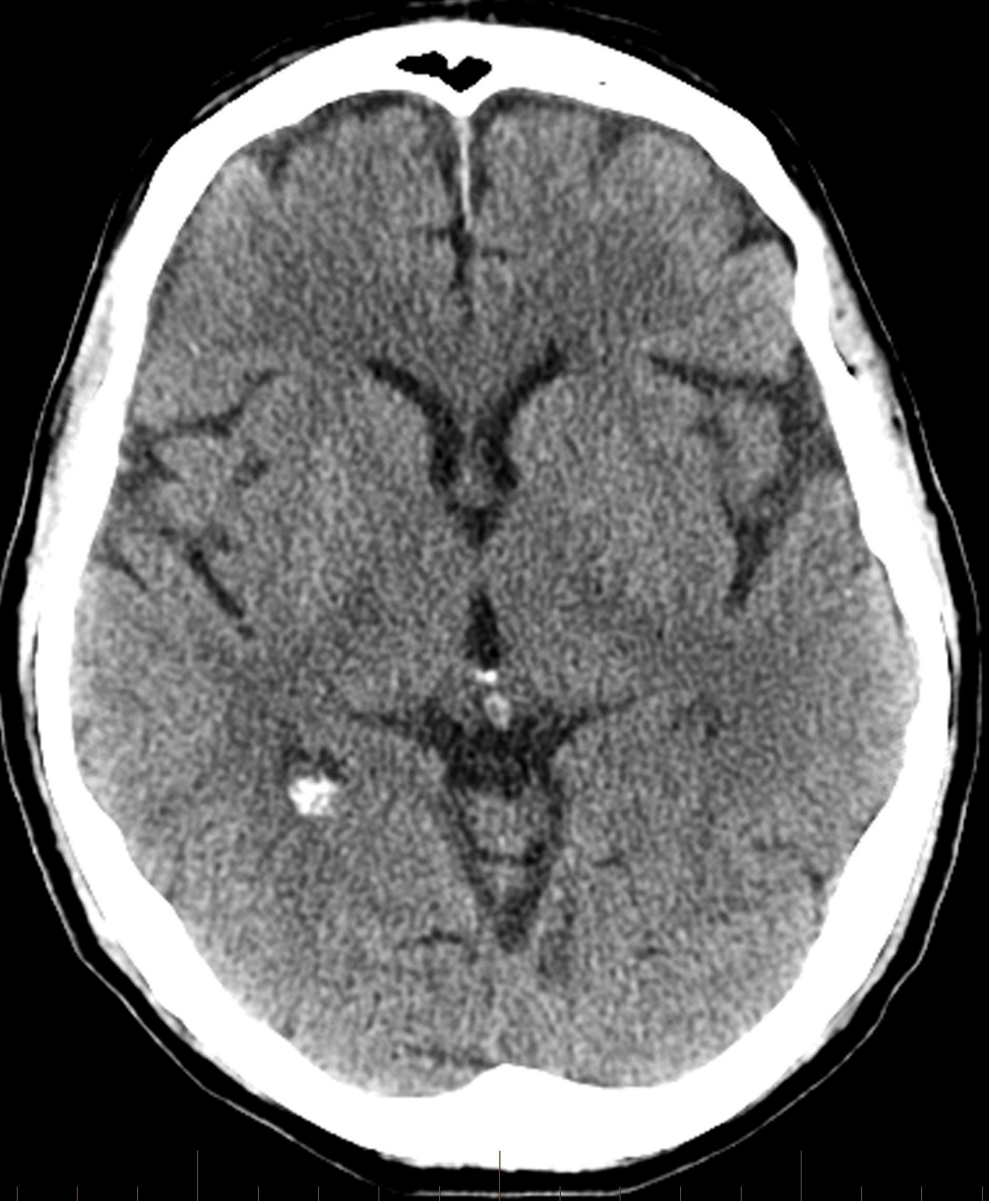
Follow-up head computed tomography performed early the next day shows no significant acute infarction or intracranial hemorrhage.
